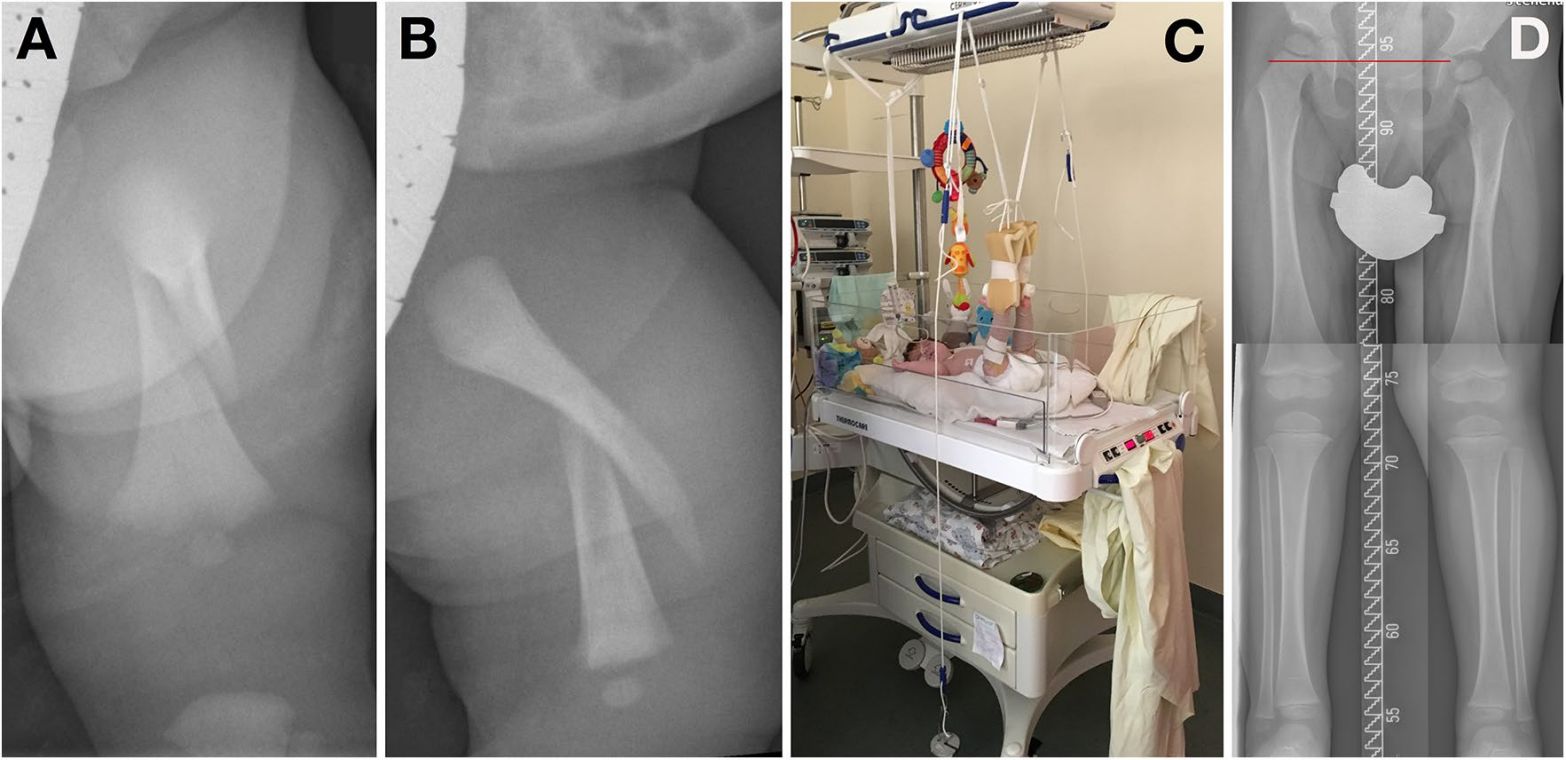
A birth-related femoral shaft fracture in a newborn: initial fracture (A, B), the newborn during skin traction (C), and radiographic outcome at the age of 2.5 years (D). The shortening of the fractured limb is 13 mm, but no restrictions on daily activities or limitations during sports have been reported
